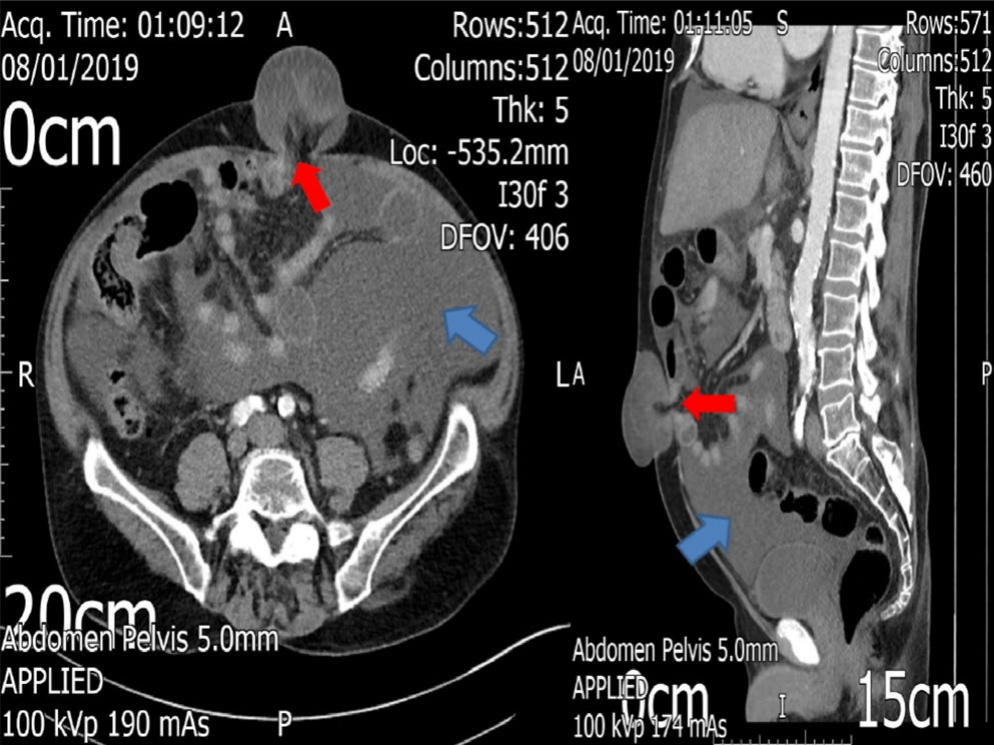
Axial and sagittal views of the CT scan prior to the initial emergency ventral hernia repair, showing the site of the incarcerated umbilical hernia, with congested small bowel protruding through the defect. Note the compression of the small bowel at the level of the constriction ring of the hernia, suggesting the development of closed‐loop obstruction (red arrows) and the presence of large‐volume, tense cardiac ascites (blue arrows)
